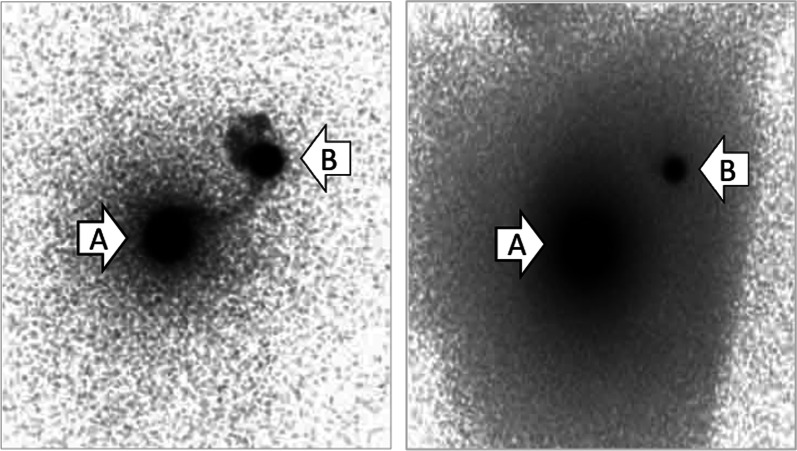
Both RI and ICG were detected in an axillary node. (RI scintigraphy, A Injection Site, B Sentinel Node)
